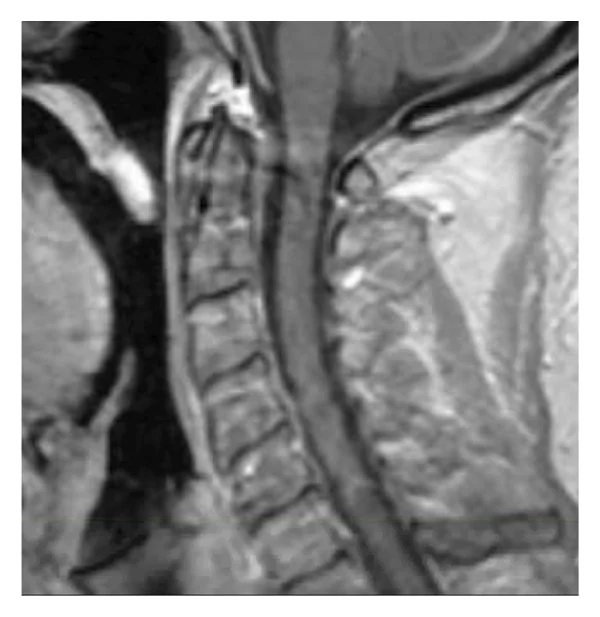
Sagittal T1‐weighted MRI of the cervical spine demonstrating no evidence of significant disc herniation, foraminal narrowing, or cord compression to account for the patient’s right upper extremity deficits.
